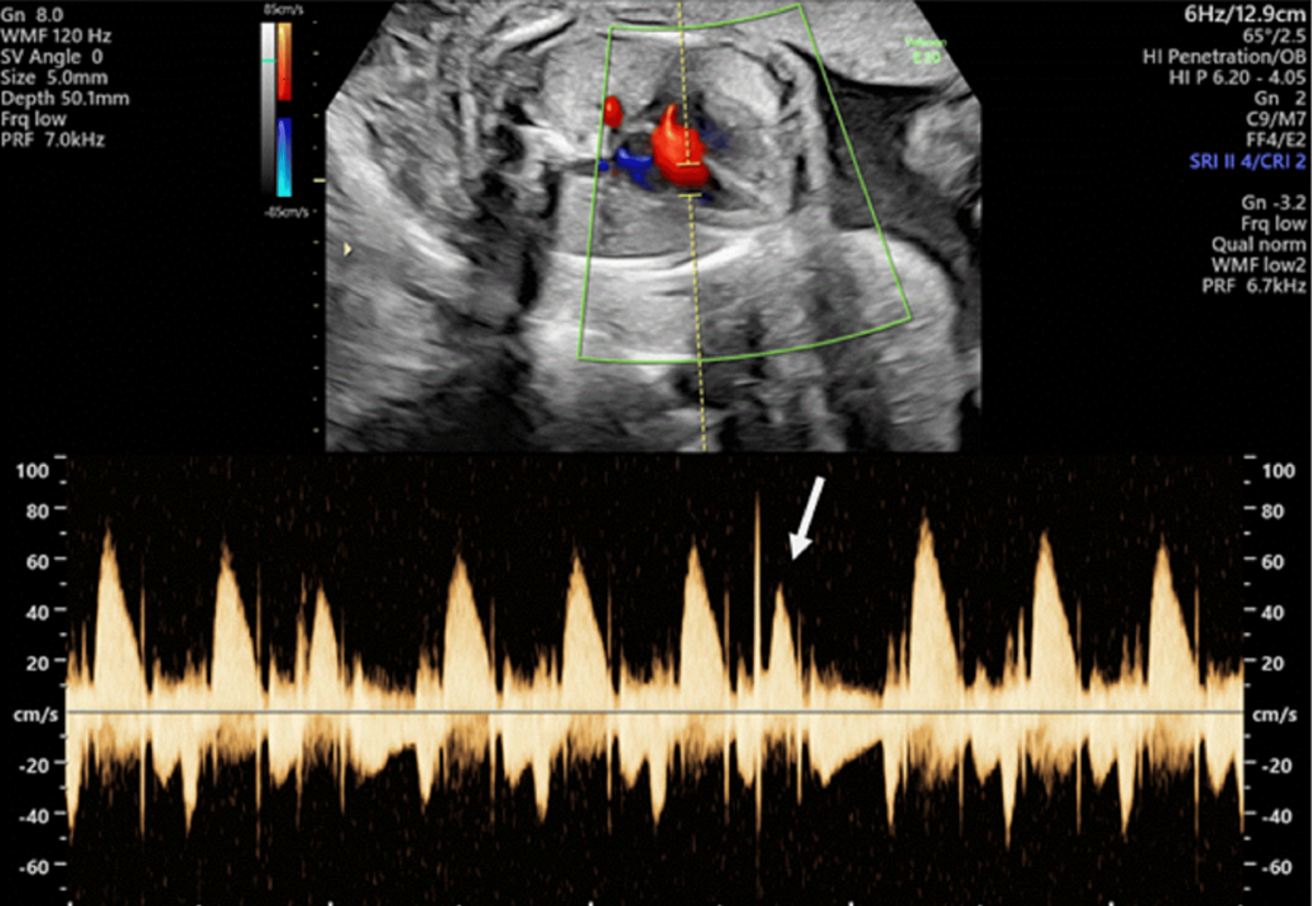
Simultaneous pulsed-wave Doppler of the mitral inflow and left ventricular outflow tract in the fetus, demonstrating a premature beat The premature beat (white arrow) is most consistent with a premature ventricular contraction when correlated with the M-mode findings in Figure 1. A compensatory pause is observed following the premature beat.
